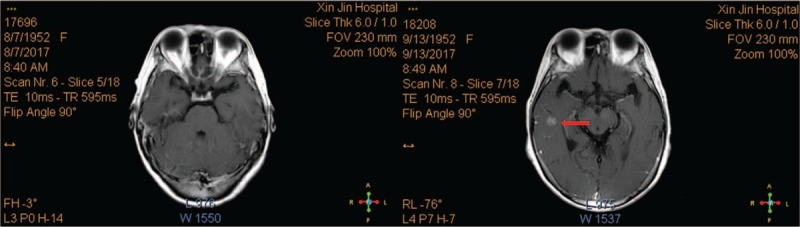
Magnetic resonance imaging shows the nodules in the brain before and after metastasis.
